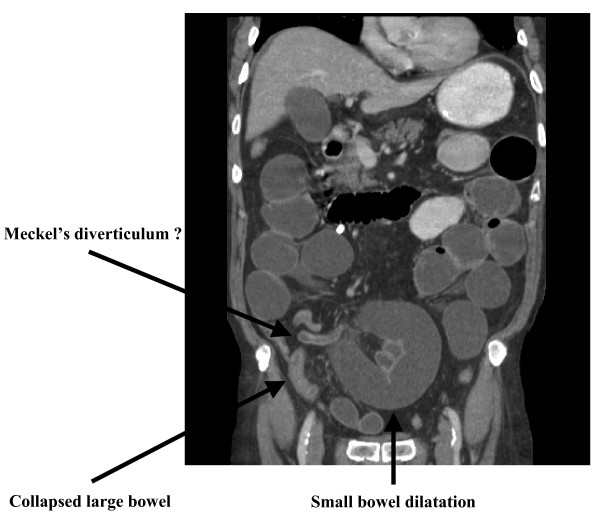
Reconstructed computed tomography coronal scan of the abdomen. This image shows small bowel obstruction as a result of a stricture in the terminal ileum. A postoperative review suggested a Meckel's diverticulum could be described.
